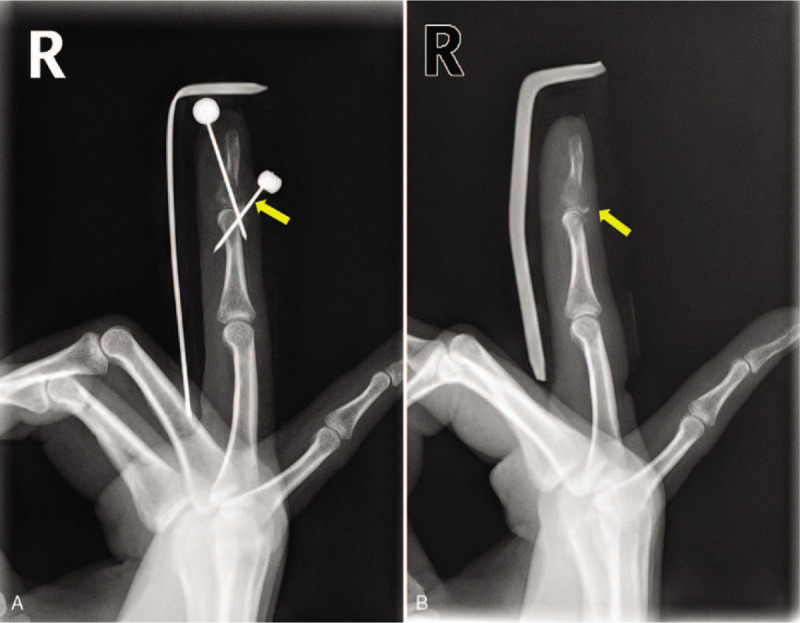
Osteolytic lesion seen on 2-wk postoperative radiographs.
